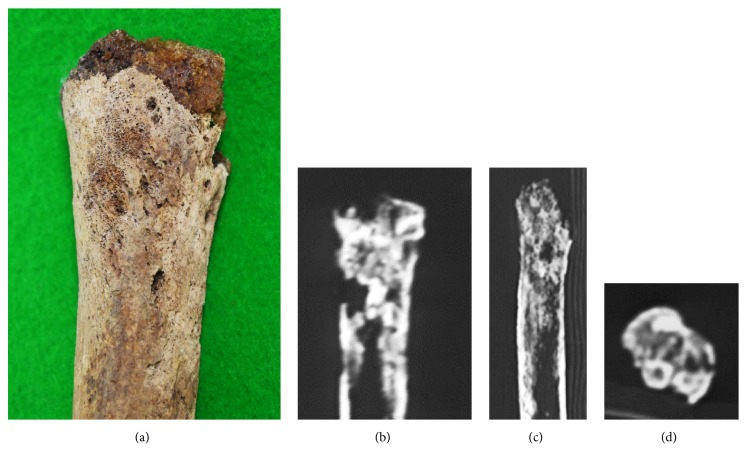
Photographs of the right humerus. Lesions showing irregular minute osteoblastic changes were observed on the anterior surface of the proximal region and bone surface of the crest of the lesser tubercle over the intertubercular sulcus (a). On CT, continuous osteosclerosis was observed from the superficial layer to the inner region in the proximal metaphysis of this humerus (b-d).
